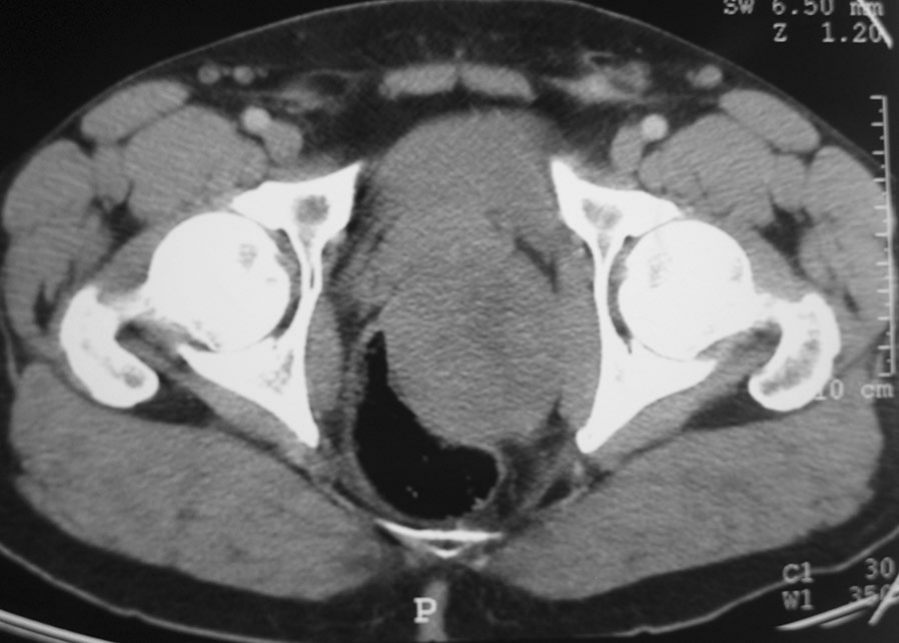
Computed tomography scan findings: axial section demonstrating a bulky and well-circumscribed mass developing in the pelvis with evident displacement of the rectum and bladder
